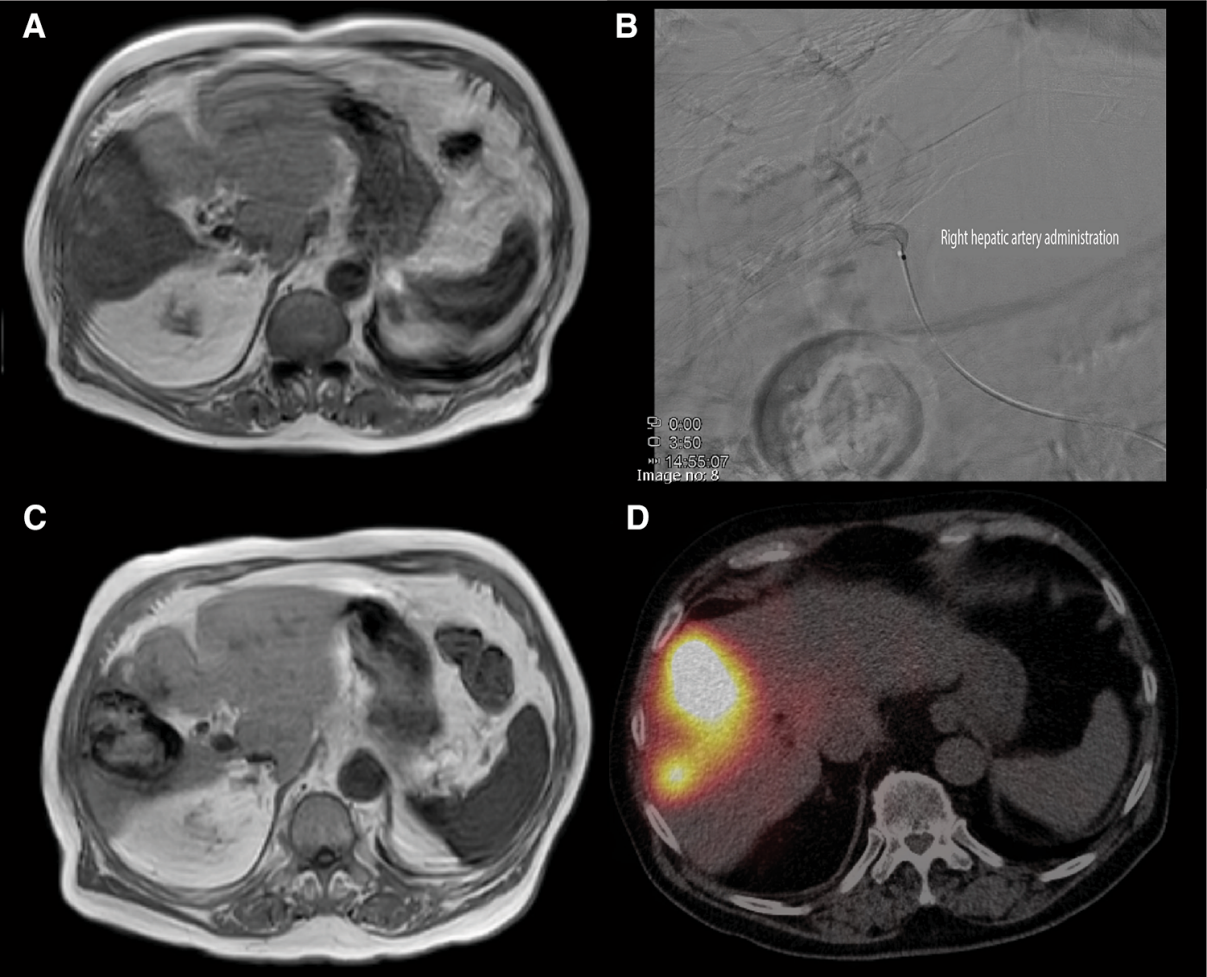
85 year old male diagnosed with hepatocellular carcinoma (HCC). At presentation, contrast enhanced T_1_ MRI (A), a solitary hypervascular lesion in segment 5, 6 and 8 with a maximum diameter of 8.1 cm was seen. At tumor board, the patient was considered for first-line SIRT. The ^166^Ho scout procedure consisted of a single injection of 233 MBq of ^166^Ho microspheres in the right hepatic artery (B) and subsequent SPECT/CT imaging showed no lung shunt, no extrahepatic deposition of activity elsewhere and visually good tumor targeting. The patient proceeded with ^166^Ho treatment in the afternoon (on the same day), in which 4.3 GBq of ^166^Ho microspheres were administered in the right hepatic artery (B). 3 months after treatment, follow-up contrast enhanced T_1_ MRI (C), showed a good response reducing its size from 8.1 cm to 5.8 cm and complete response according to mRECIST. Post-treatment SPECT/CT (D) 3 days after treatment confirmed the planned high accumulation of particles in the lesion, without extrahepatic deposition of activity (and no lung shunt). At this moment, more than 3 years after treatment, the patient has no signs of recurrent disease on imaging
